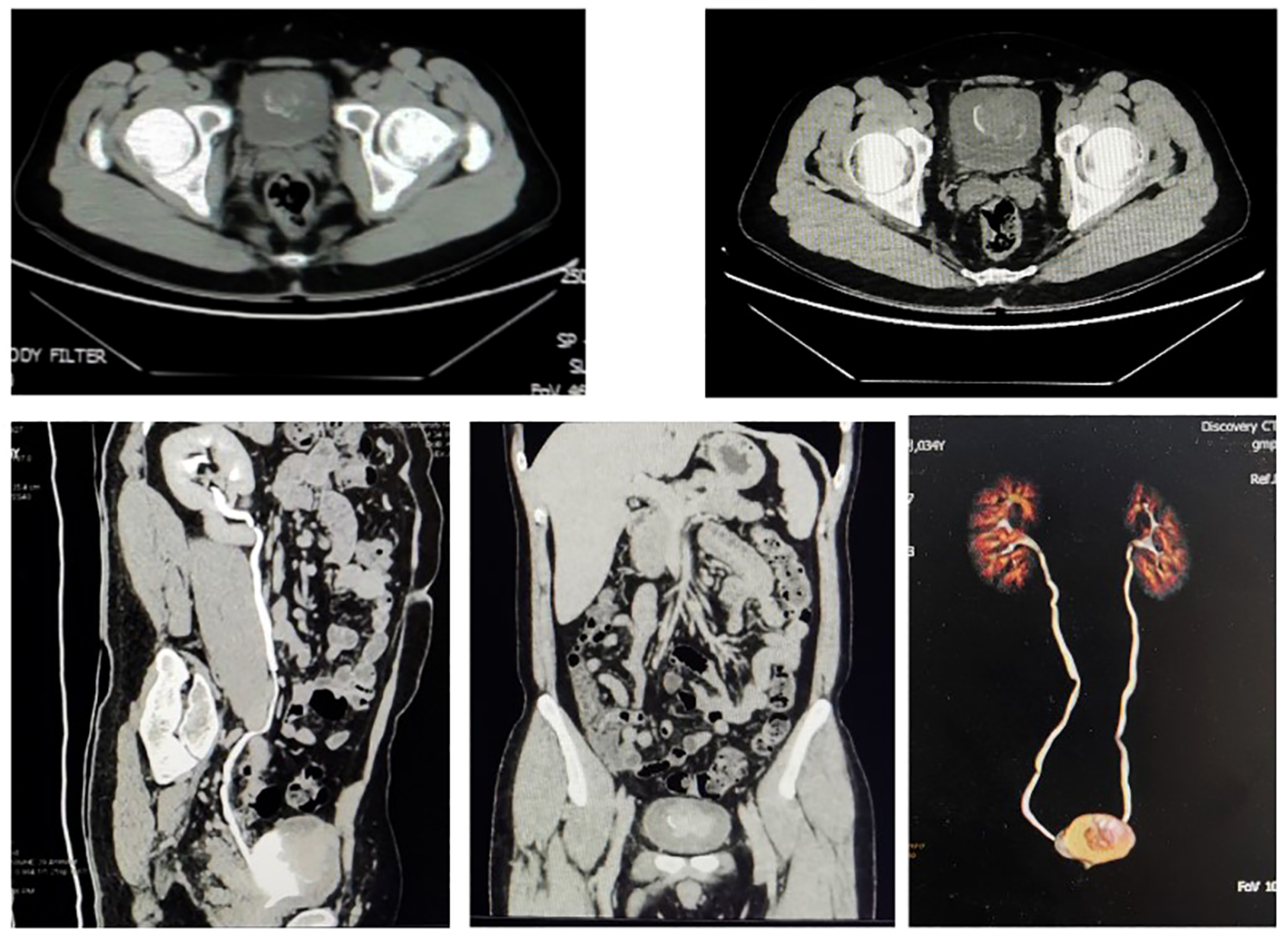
CT scan of the abdomen with contrast reveals the bladder tumor.
